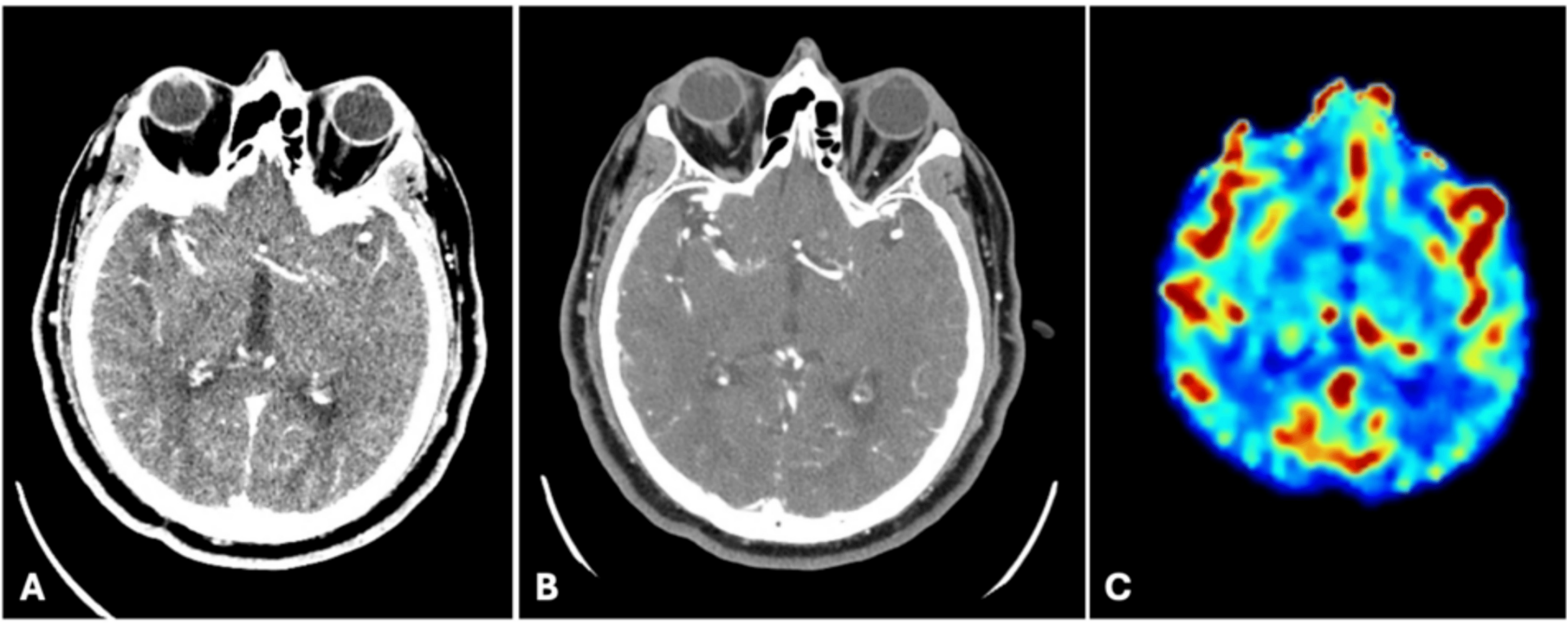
Postoperative CT imaging Non-contrast head CT (A), CT angiogram of the head (B), and CT perfusion (C) demonstrated no acute findings and no perfusion deficits immediately following first sign of neurological deficit.
